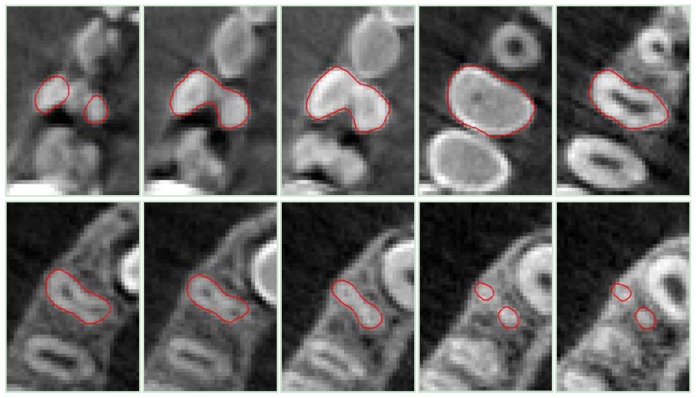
Results of the DRLSE method for *tooth 14*, obtained by processing some CBCT slice images.
